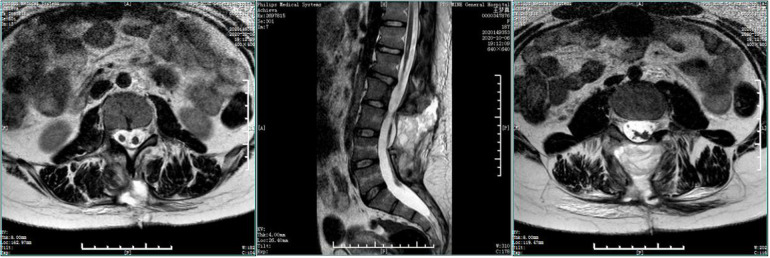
Postoperative MRI. The compression of the spinal cord was removed, with diastematomyelia and tethered cord, without fusion of the L4-5 vertebral arches.
